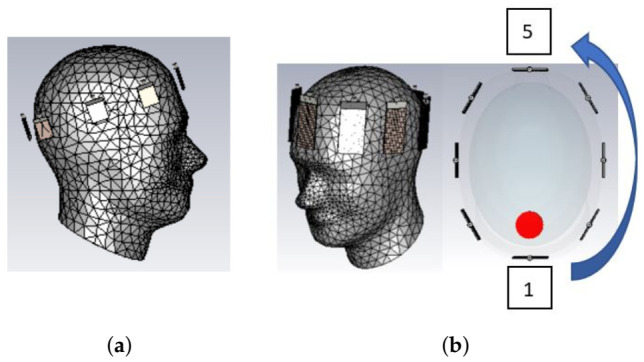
(a) 8-PSMA array (“System 1”) (b) 8-MTS-Enhanced PSMA array (“System 3”) and its transversal view. “System 2” is equal to “System 2” except for including non-enhanced PSMAs.
